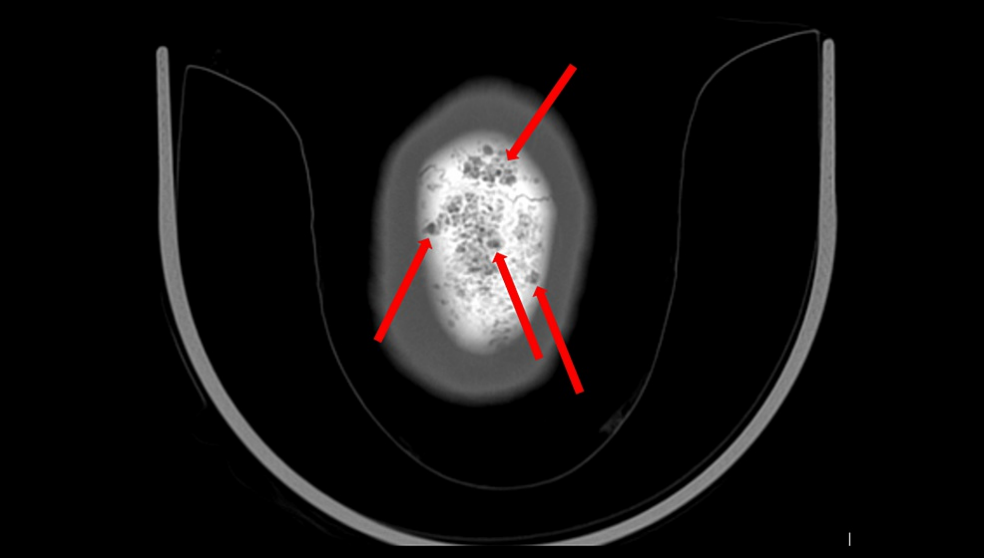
Extensive lytic appearance throughout the calvarium on CT CT, computed tomography
